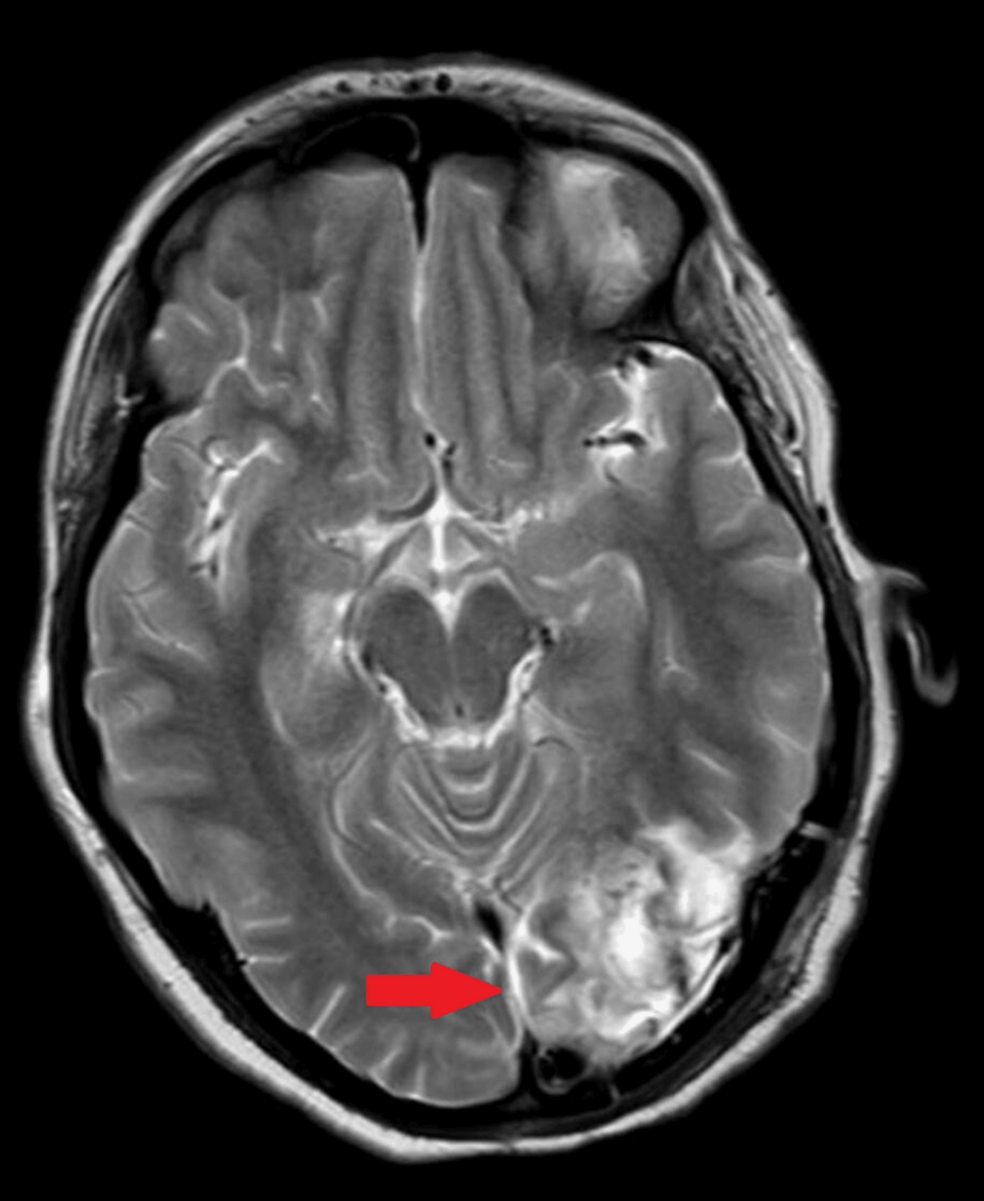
Postoperative axial T2-weighted MRI brain scan showing parenchymal changes in the left occipital region consistent with post-surgical effects (red arrow).
